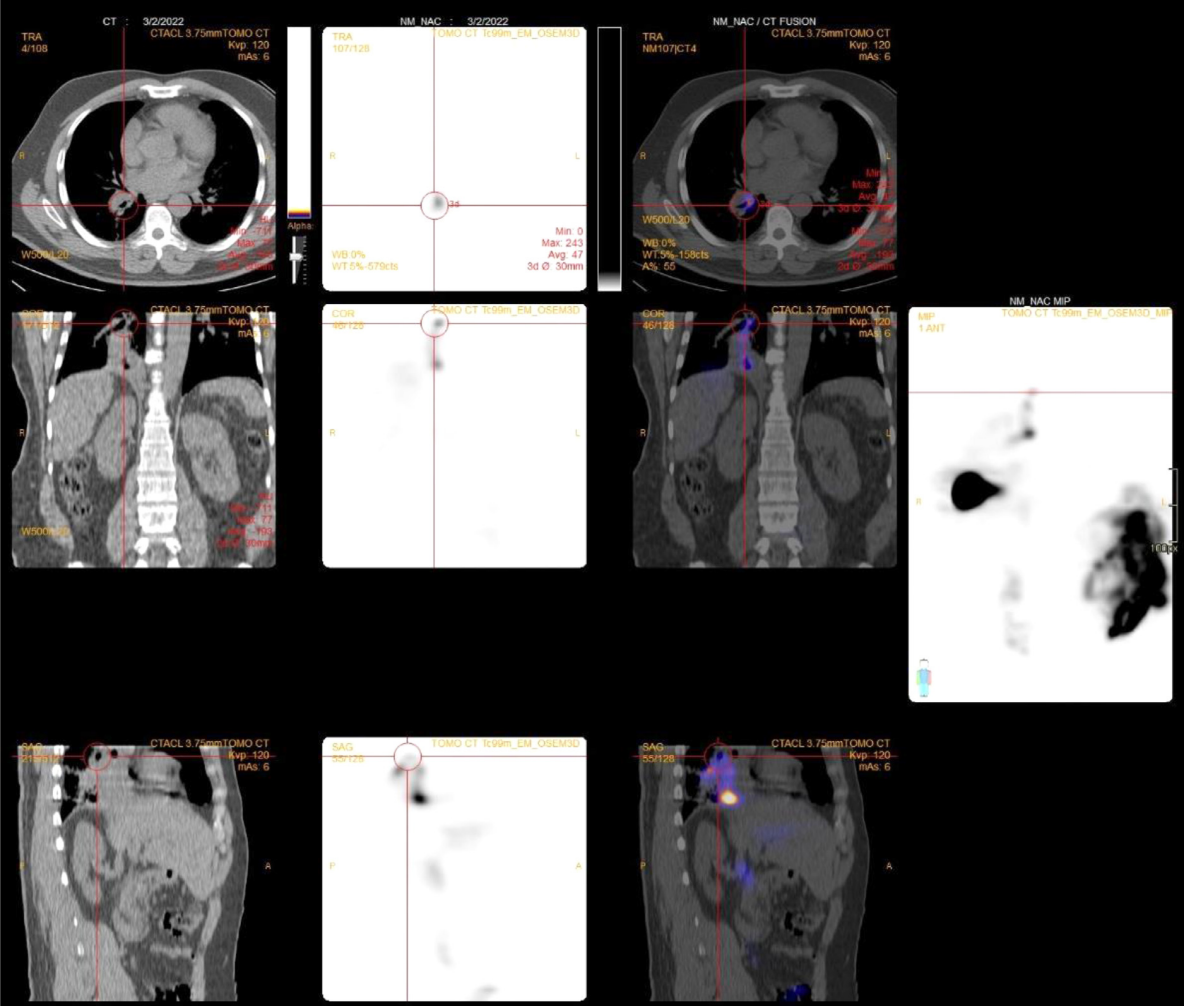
Technetium-99m (Tc-99m) HIDA scan and SPECT/CT scan after 1 hour after injection of radiotracer show tracer passage cranial to the right lower lung, findings confirmed the biliary uptake.
